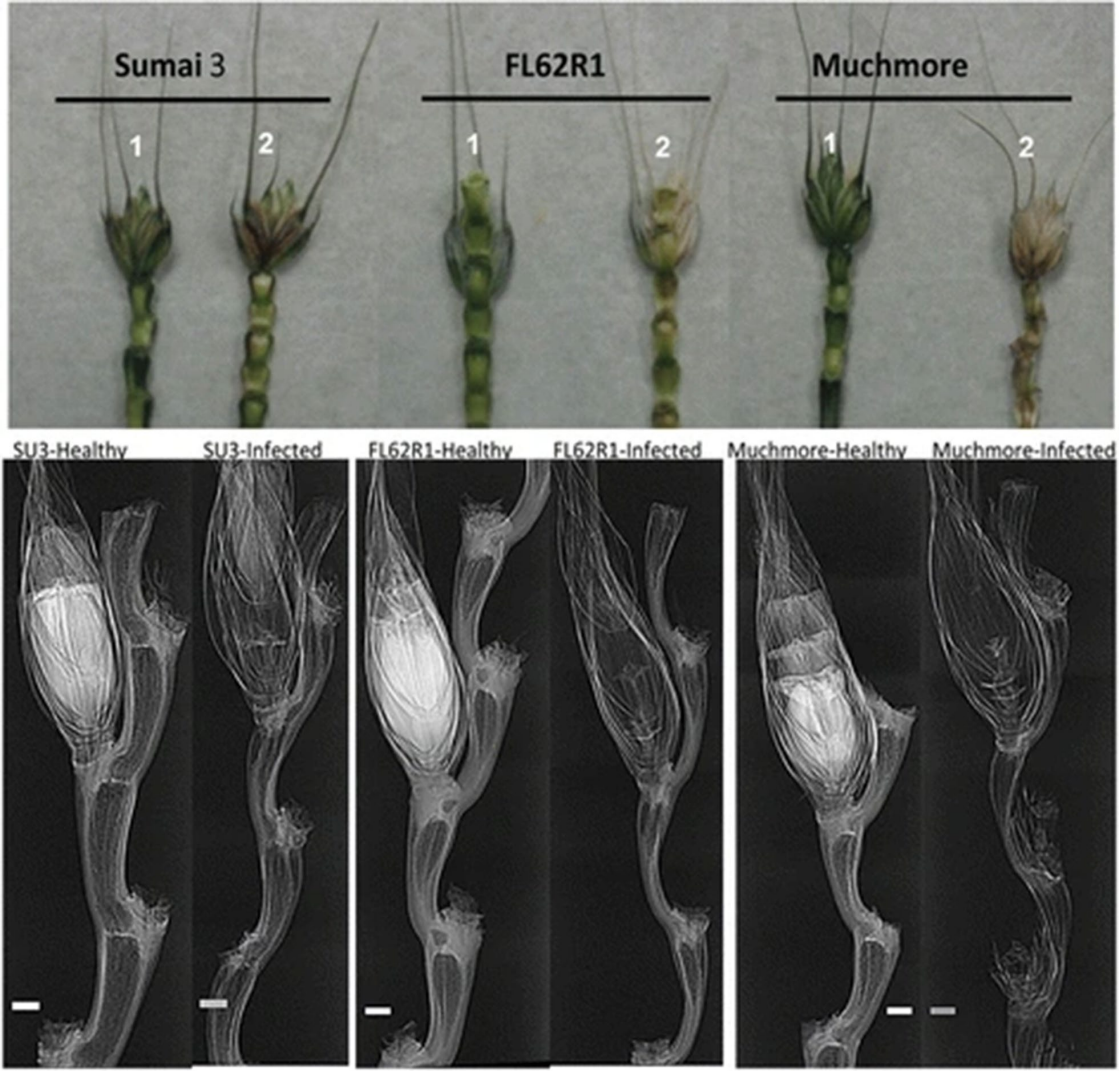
Phase contrast X-ray images of healthy (1) and infected rachis (2) of wheat cultivars at four days after inoculation with FHB [90]
